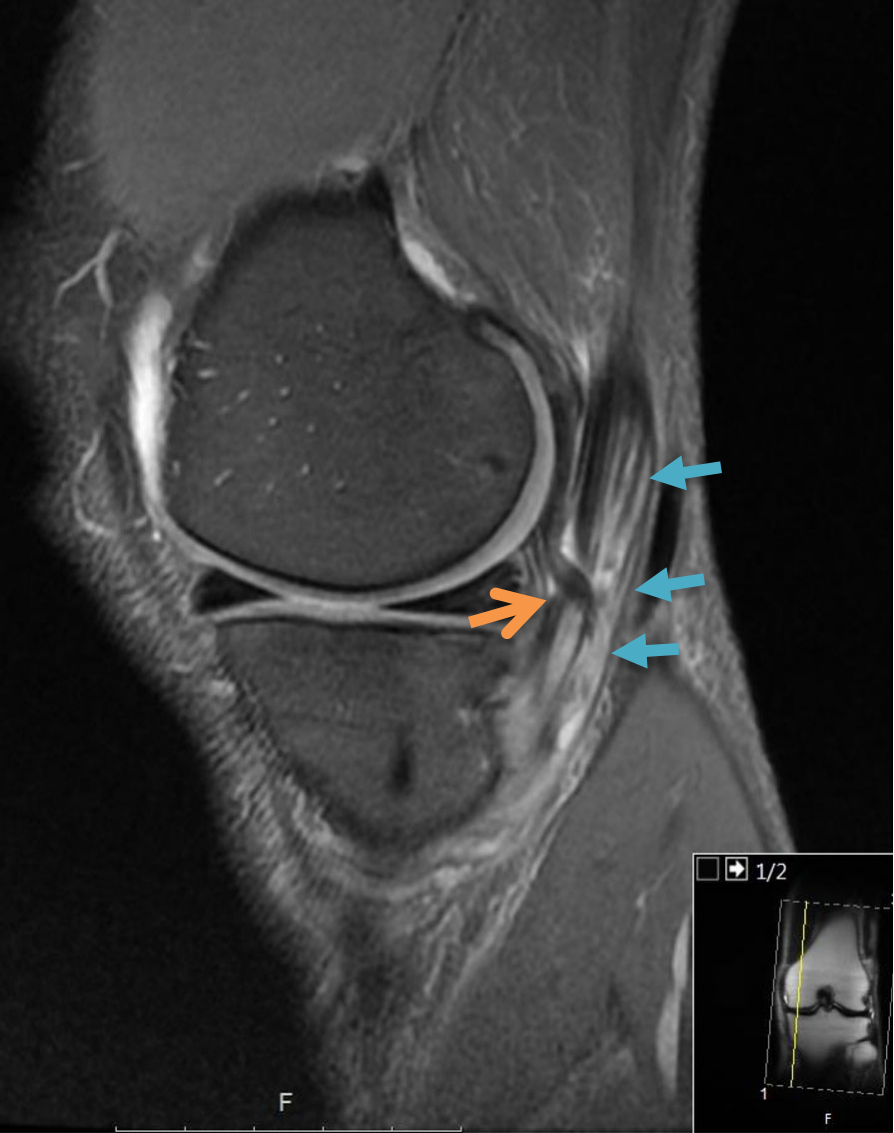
Sagittal magnetic resonance imaging of the right knee, depicting the cord-like structure (*orange arrow*), originating from the fabella, impinging on the semimembranosus tendon (*blue arrows*)
